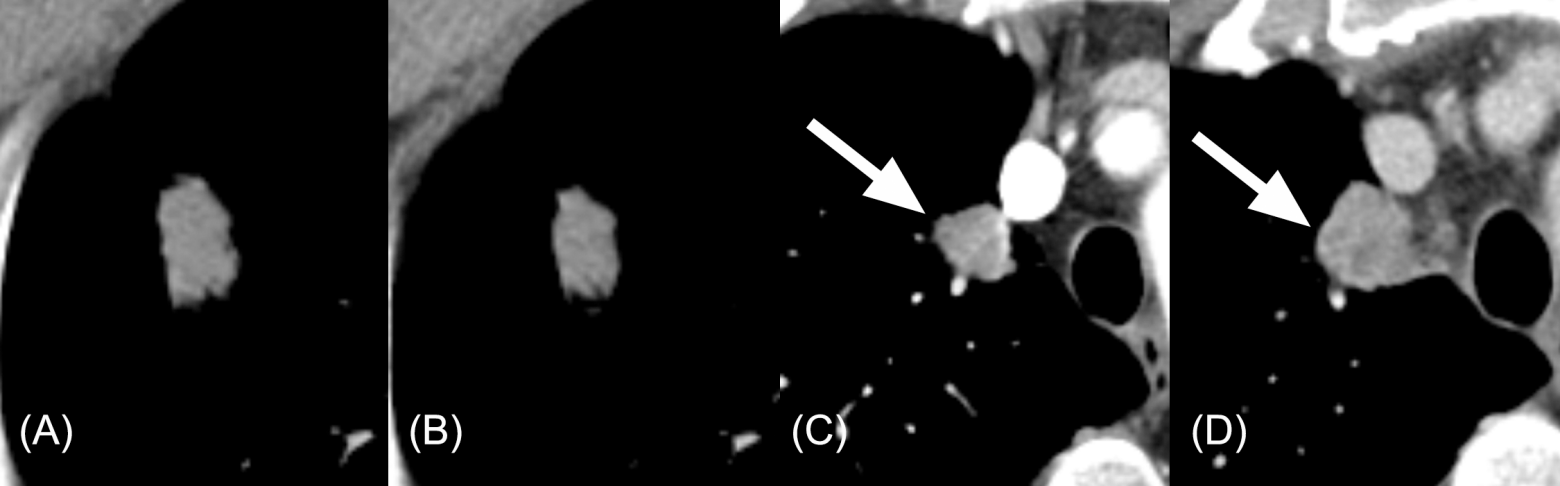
Radiomic features and their association with progression-free survival (PFS). (A) On the baseline CT, roundness of the tumor was 0.7352 and grey-level nonuniformity was 23.06. (B) On the first follow-up CT (9 weeks later), roundness was 0.7355 (percent change: 0.0422%) and grey-level nonuniformity decreased to 16.93 (percent change: -26.57%). PFS in this patient was 23.6 months. (C) For this patient, whose PFS was only 1.4 months, tumor roundness was 0.8591 and grey-level nonuniformity was 24.13 on the baseline CT. (D) On the first follow-up CT (9 weeks later), grey-level nonuniformity increased to 43.11 (percent change: 78.63%), while the roundness was 0.8040 (percent change: -6.411%).
